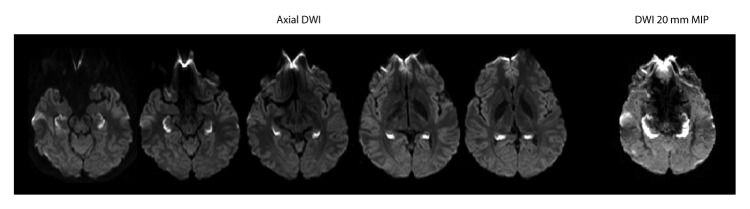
Diffusion-weighted imaging (DWI)* findings at initial brain MRI in a patient with unusual amnestic syndrome — Massachusetts, 2012 Abbreviations: MIP = maximum intensity projection; mm = millimeter; MRI = magnetic resonance imaging. * Axial DWI demonstrates bright signal consistent with complete bilateral hippocampal ischemia. The complete extent of hippocampal ischemia is best evident on thick 20 mm MIP images constructed from the axial DWI data.
